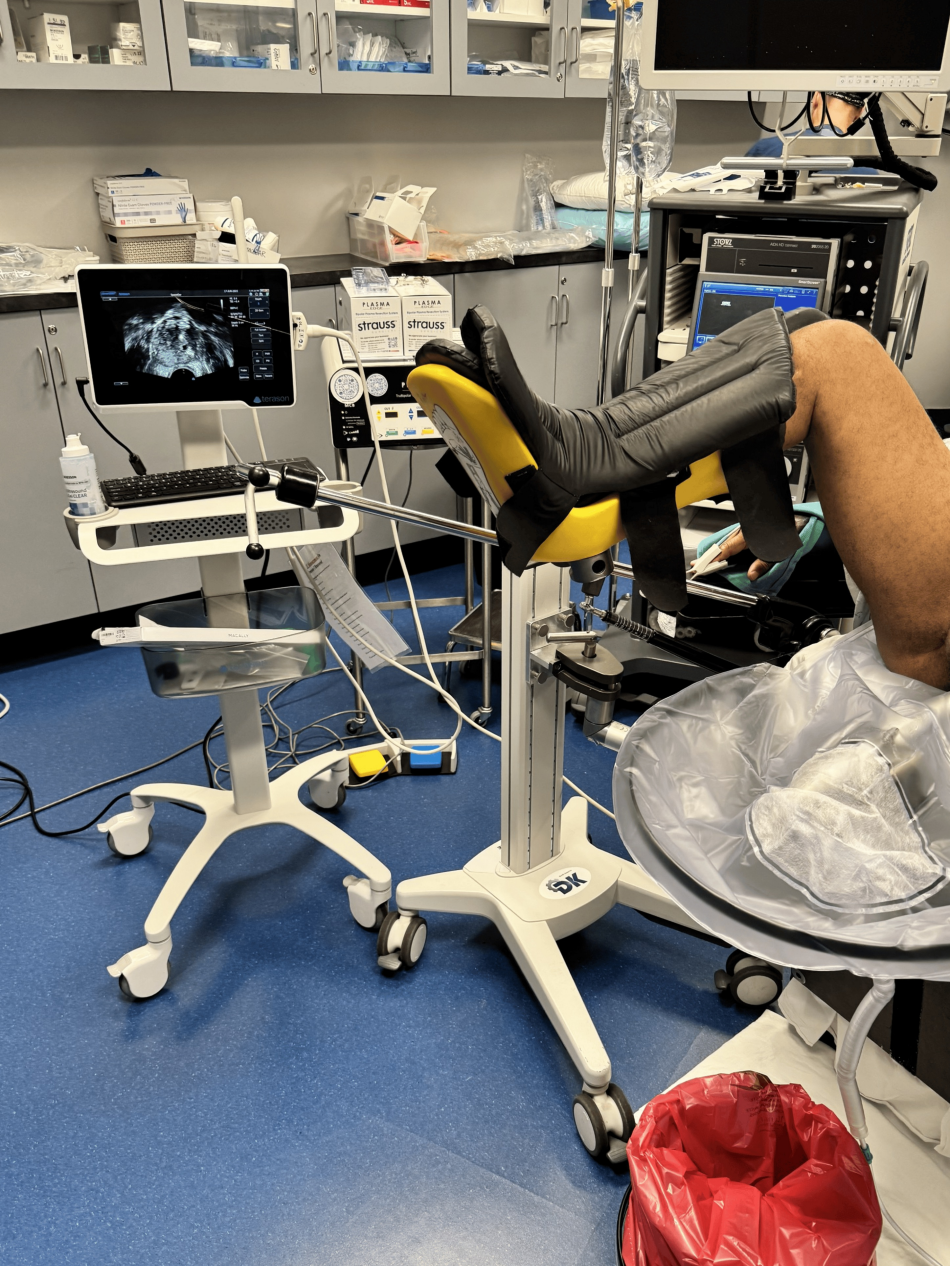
Operating room setup. Stepper-mounted transrectal ultrasound adjacent to the operative field with the resectoscope tower aligned to the surgeon. Source: Authors; no copyrighted material used; permission not required.
